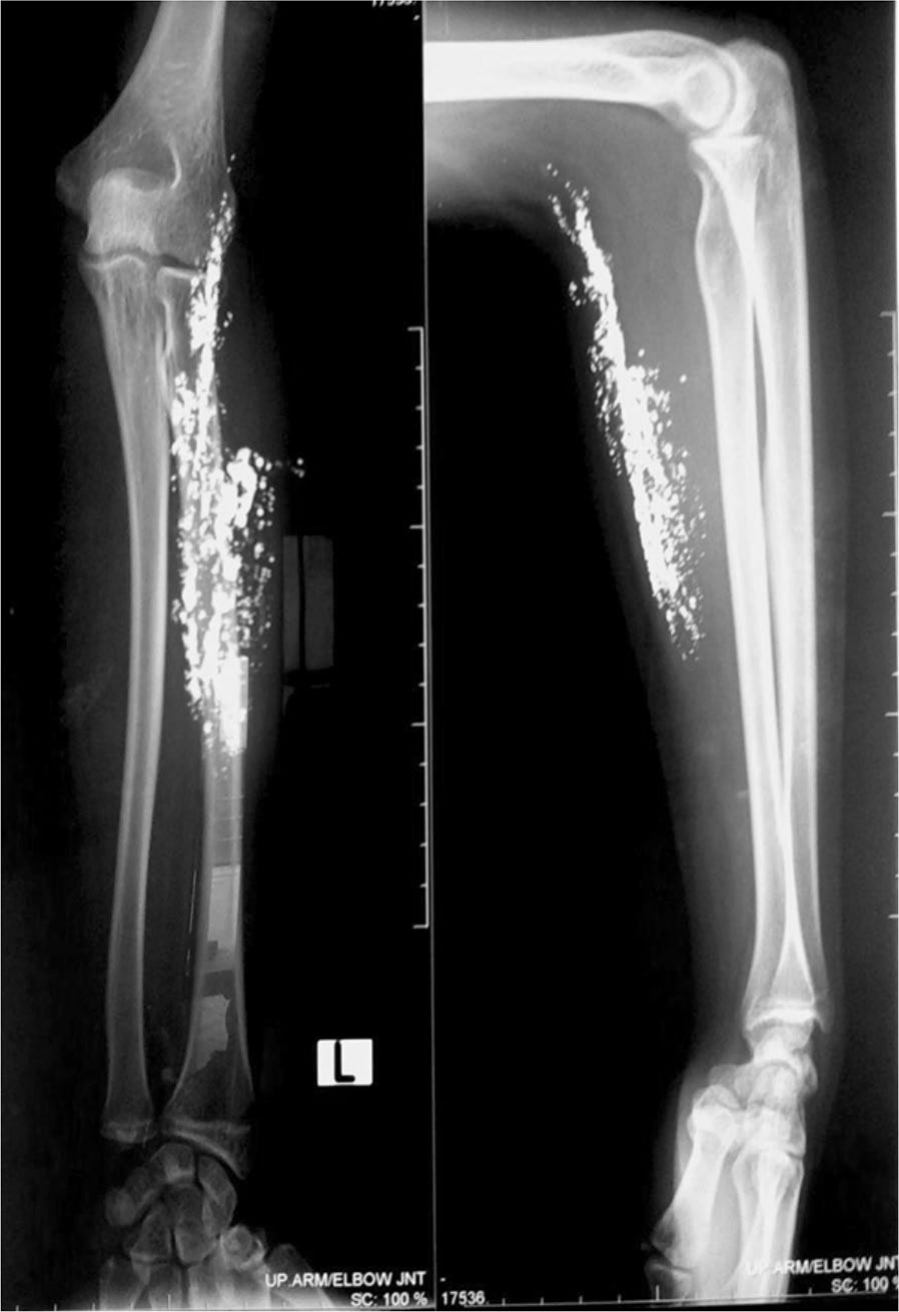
x-rays of hands showing deposition of mercury in subcutaneous and deep tissues
